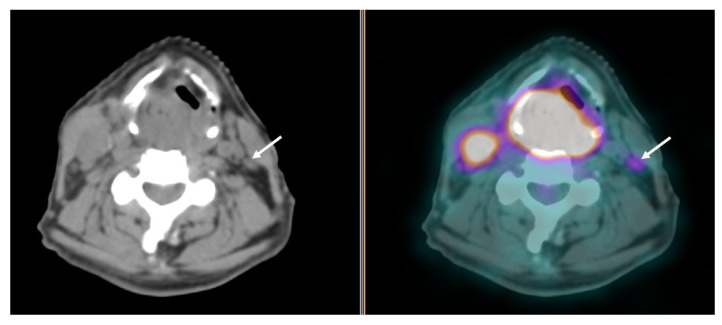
PET/CT images showing intense ^18^F-FDG uptake in a large hypopharyngeal cancer and an enlarged lymph node on the right cervical side; another sub-centimetric cervical lymph node with a mild increase in ^18^F-FDG uptake is seen contralaterally (arrows).
